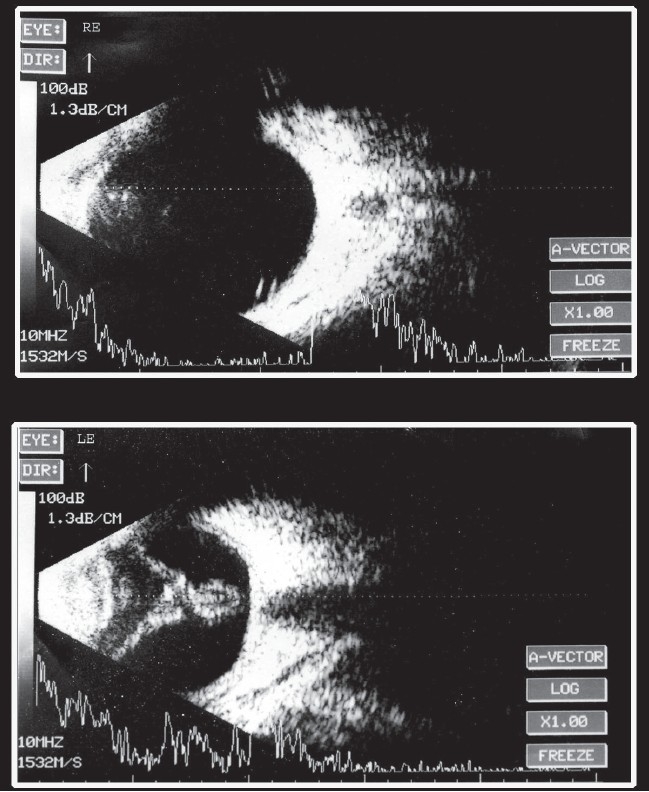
Ultrasonogaphy B-scan of both eyes showing no abnormality in the right eye whereas the left eye shows presence of longstanding closed funnel retinal detachment
